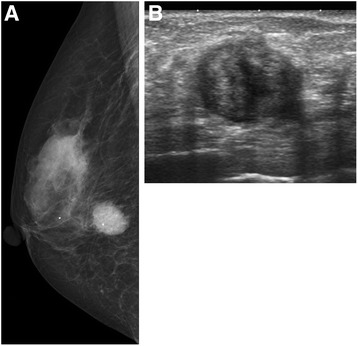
Imaging findings. a Mammogram shows a round, microlobulated mass. b Ultrasonogram shows a hypoechoic solid mass with an irregular margin
